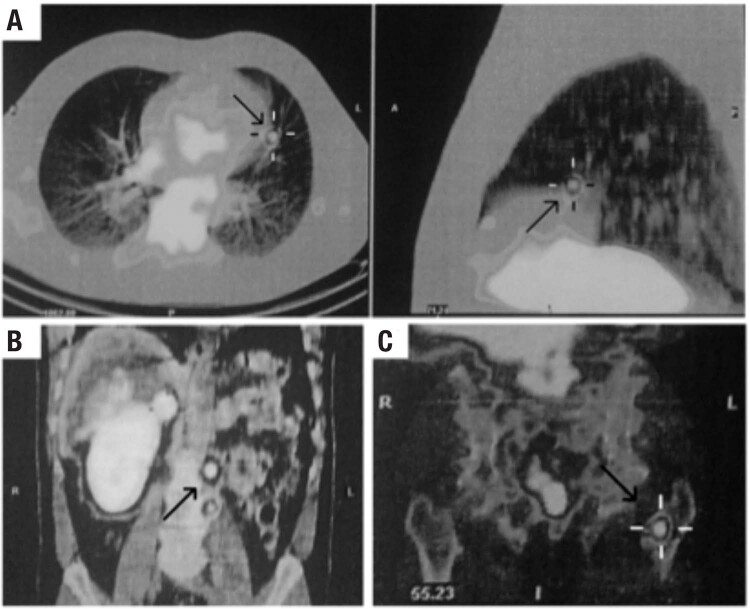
^177^Lutetium-Octreotate Single-Photon Emission Computed Tomography (SPECT) revealing areas of radiopharmaceutical hyperconcentration (shown by black arrows) in the lungs (A, axial and sagittal view), left para-aortic lymph nodes (B, coronal view), and left femoral intertrochanteric region (C, coronal view).
